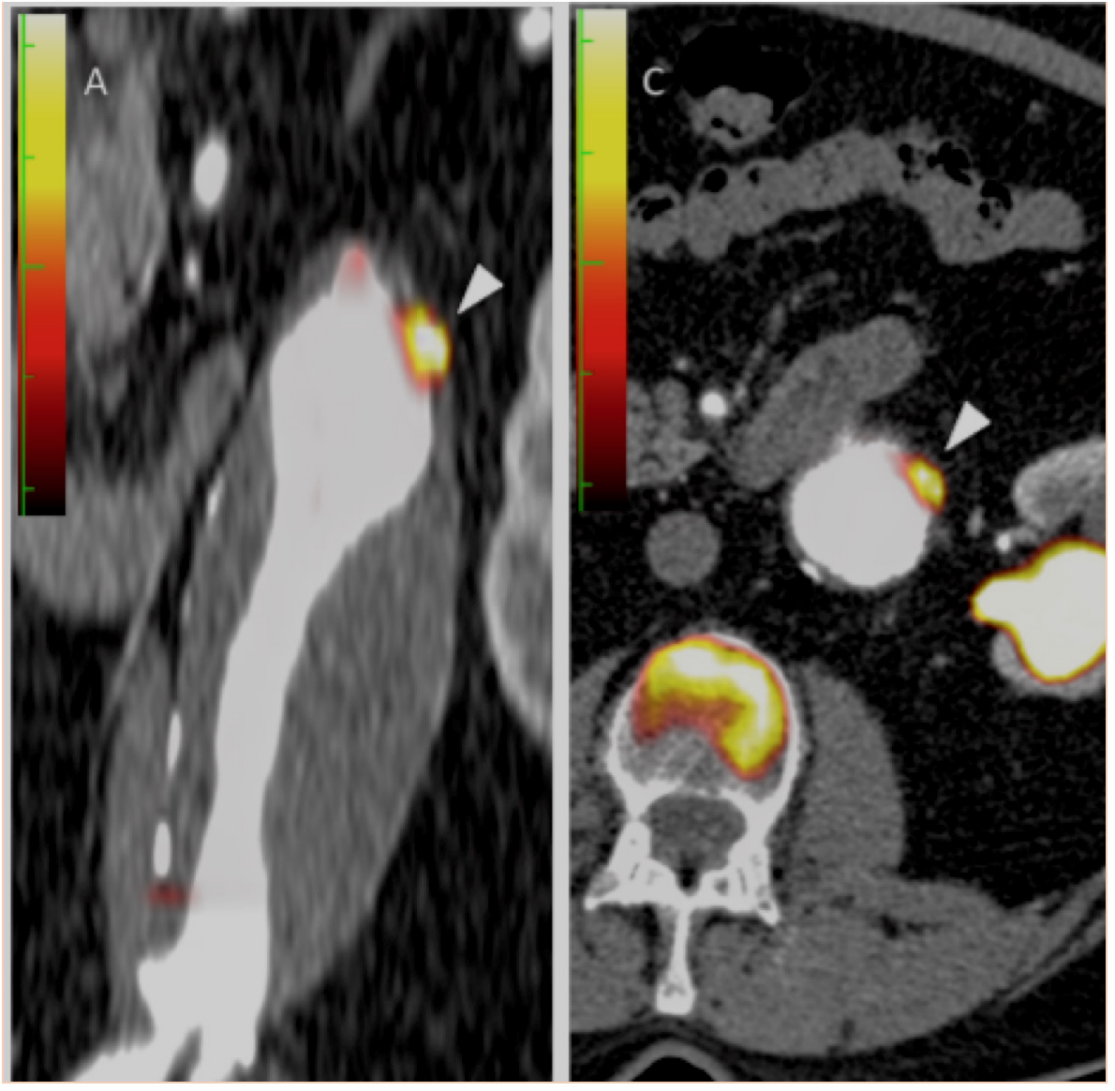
Coronal and transaxial fused positron emission tomography and contrast CT images demonstrating focal 18F-FDG uptake within the aneurysmal wall (white arrowheads). Note also the calcified lateral aspect of the aneurysm.
